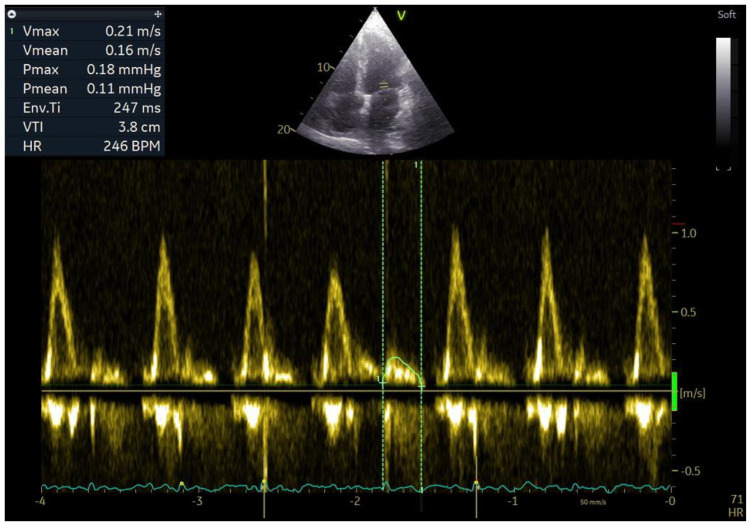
Left atrial fibrillatory contraction flow (Afc). Pulsed-wave Doppler of mitral filling in the apical four-chamber projection during atrial fibrillation. Velocity time integral (VTI) and AFc velocity measurement. AFc VTI 3.8 cm. AFc V 21 cm/s.
